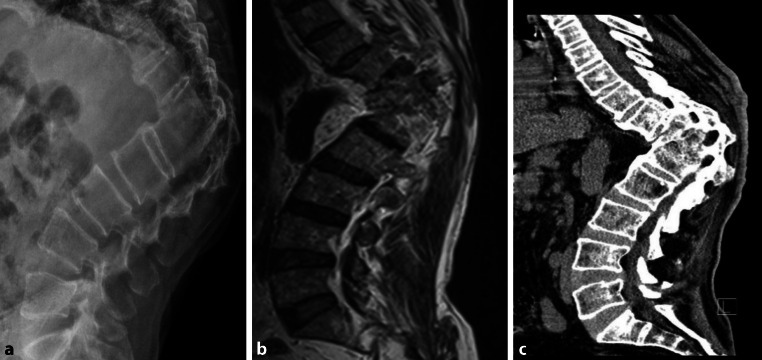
This 69-year-old male patient in 2020 was in inpatient care with a fracture of vertebral bodies due to tuberculosis infection. A 90° gibbus in the area of the thoracolumbar transition TH12–L1 with ankylosis and a Cobb angle of 45° is visible. a Conventional X‑ray. b Magnetic resonance imaging. c Conventional computed tomography
